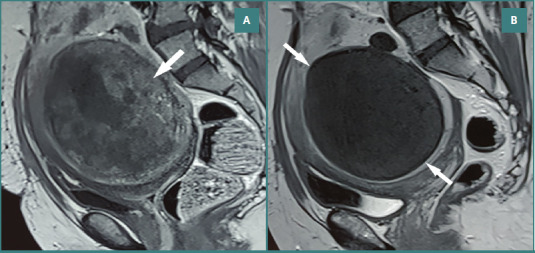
Uterine fibroid before and after uterine artery embolization. A, Pre-embolization sagittal contrast-enhanced T1-weighted MRI of the uterus showing approximately 50% enhancement of a large intramural myoma. B, Post-embolization sagittal contrast-enhanced T1-weighted MRI showing absence of enhancement consistent with complete infarction.
